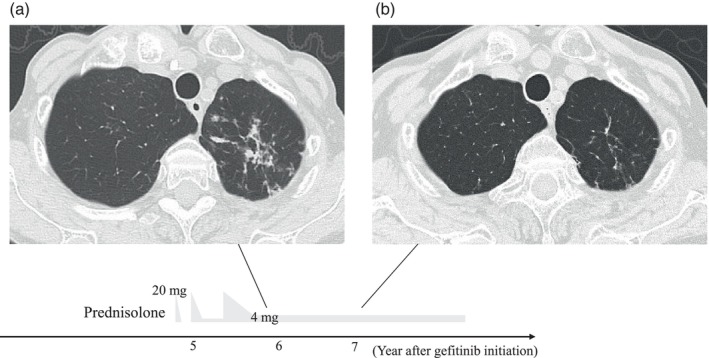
Chest computed tomography image. (a) Multiple nodular shadows in the upper lobe of the left lung at the diagnosis of tuberculosis (approximately 3 years after the initiation of osimertinib). (b) Disappearance of multiple nodular shadows in the upper lobe of the left lung after tuberculosis treatment (1 year after the initiation of antituberculosis drugs).
